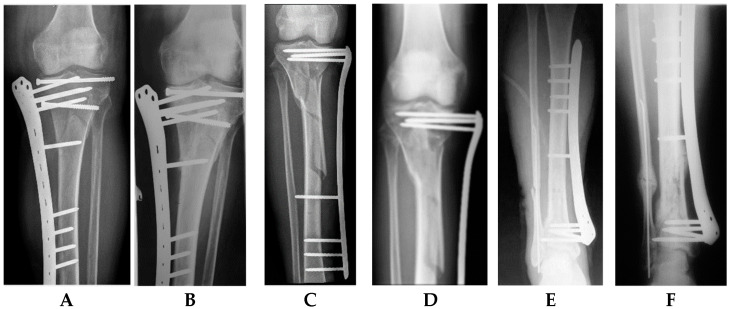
Post-operative (A,C,E) and final follow-up (B,D,F) radiographs of patients with an AO/OTA 41-C2.2 proximal tibial fracture with simple intra-articular involvement of both tibial condyles without intra-articular comminution and impaction (radiographs A,B), an AO/OTA 41-A3.1 multi-fragmentary proximal tibial extra-articular fracture (radiographs C,D), and an AO/OTA 43-A2.3 distal tibial multi-fragmentary meta-diaphyseal fracture (radiographs E,F), all accompanied by significant soft tissue injuries and treated via single-stage externalized locked plating with LISS-DF.
